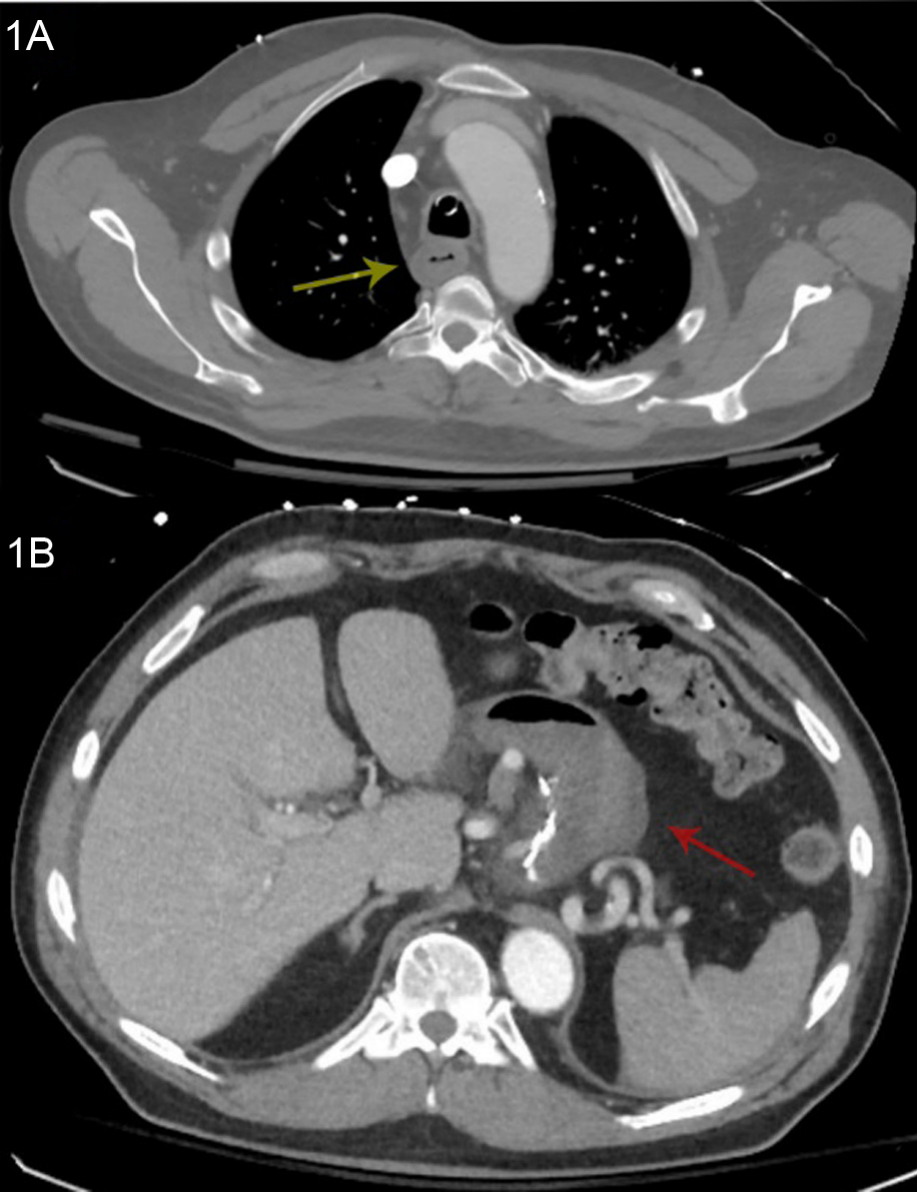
Computed tomography (CT) of the chest and abdomen shortly after admission. 1A: CT chest with contrast shortly after intubation; the yellow arrow indicates region of esophageal thickening in the proximal esophagus. 1B: CT abdomen pelvis with contrast shortly after intubation; the red arrow shows thickening of the stomach.
